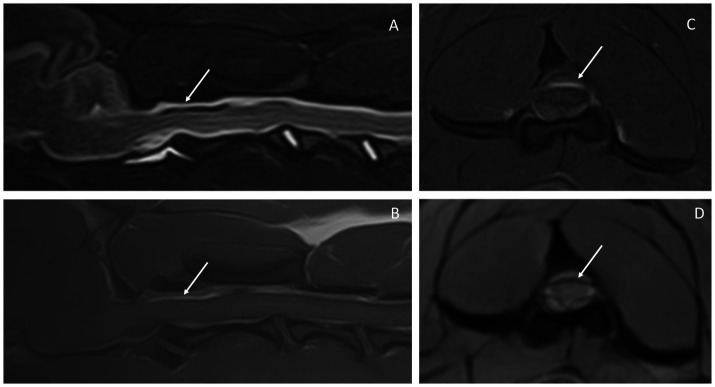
Magnetic resonance images of the reported case. Sagittal STIR (A), sagittal T1W pre-contrast (B), transverse T1W FATSAT post-contrast (C), and transverse T2* (D) at the level of C2. The arrows point out the subarachnoid space, which is heterogeneous in all sequences, showing mixed signal intensity on STIR (A) and hyperintensity in T1W (B). The latter is seen clearly in C, in which the epidural fat is suppressed and the hyperintensity within the subarachnoid space is highlighted. This same area corresponds to signal void in T2* (D).
